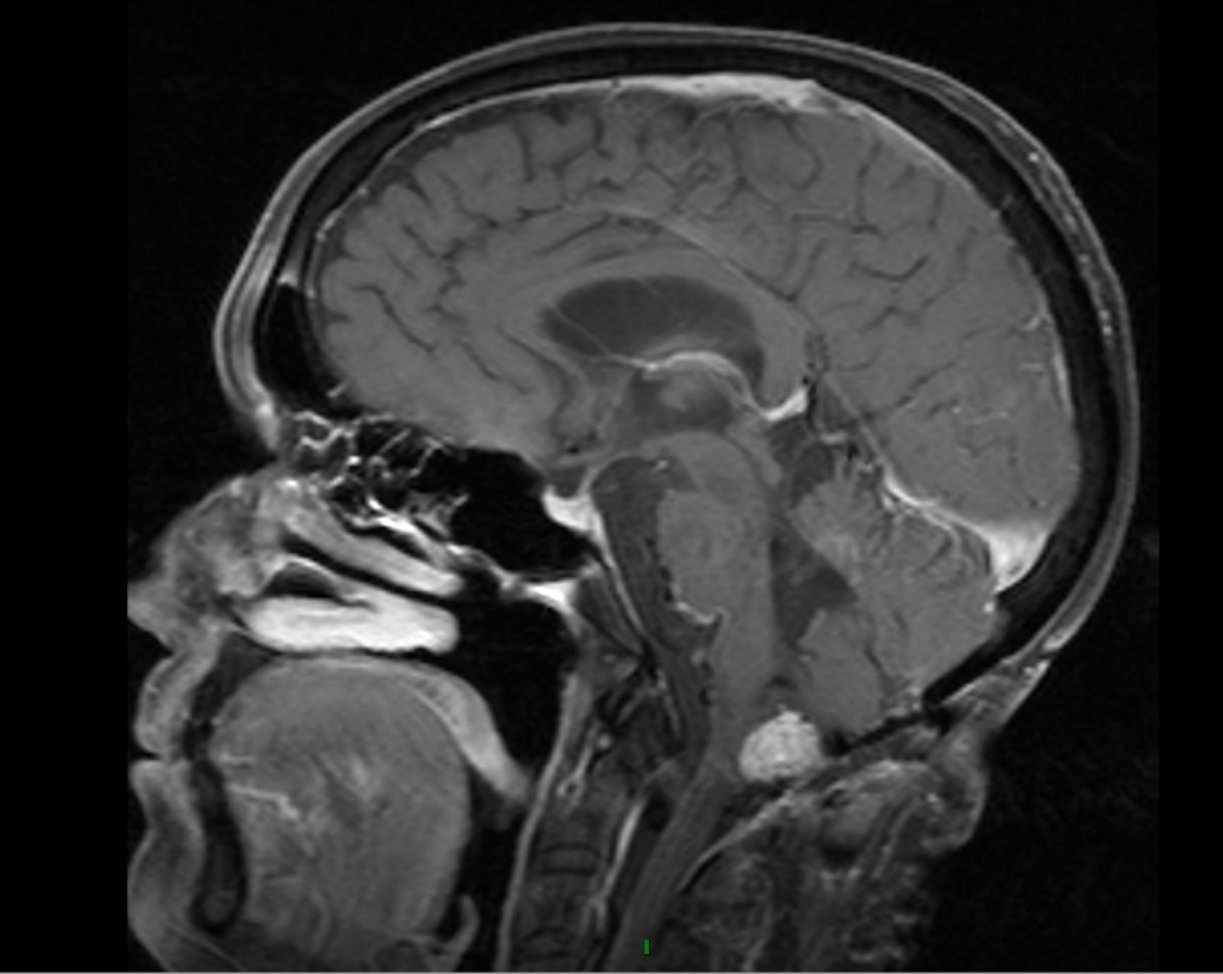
Regression of Tumor MRI of the brain showing decrease in size of patient’s hemangioblastoma at last follow-up.
